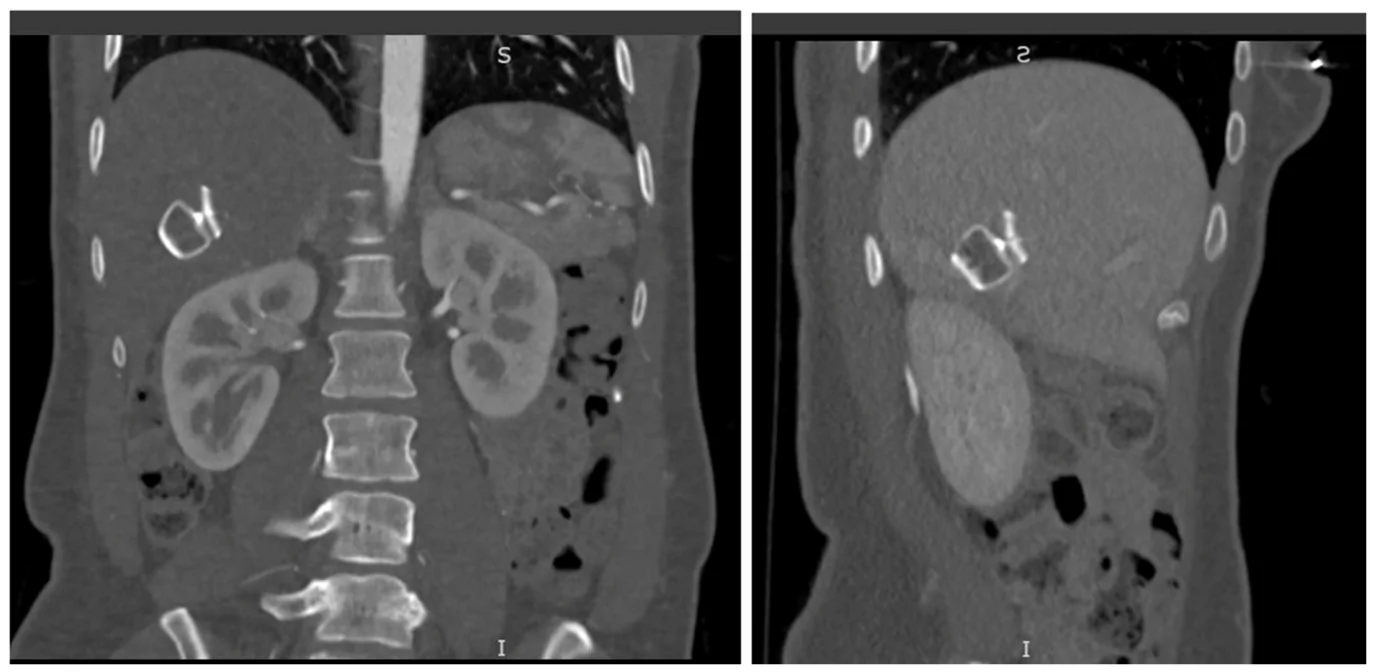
CT imaging three months after the procedure showing vascular plugs still in place with successful occlusion of the portal venous fistula.
